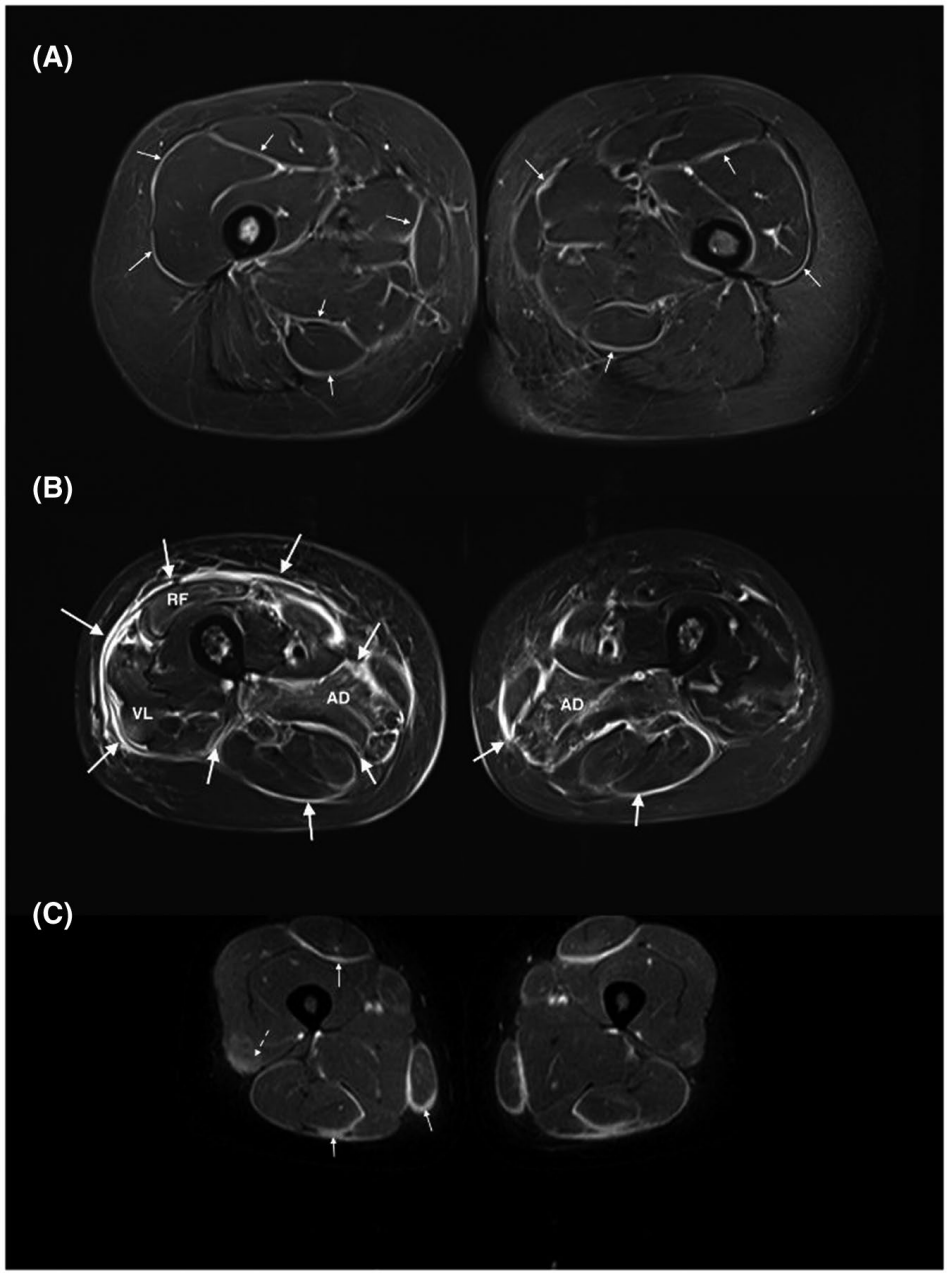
High fascia T2 signals in myositis: (A) patient with an eosinophilic fasciitis displays a diffuse hypersignal of the deep fascia and intermuscular septa (small arrows). The pelvic muscles (i.e., gluteus muscles) are also affected. (B) MRI of a patient with a graft versus host disease involving the fascia (big arrows) (diffuse hyperintensity and thickening of the deep fascia and intermuscular septa) and the muscles (especially both the adductor magnus muscles and the right quadriceps muscle). (C) ASyS patient dysplaying a fasciitis with hyperintense, thickened fascia and intermuscular septa on both sides with symmetrical distribution (full arrows). In addition, presence of a mild myositis attested by a blurred, slight T2 hyperintensity in the quadriceps muscles (dashed arrows). All pictures show thigh muscles MRI images (axial plane, T2 STIR w. seq). RF, rectus femori; VL, vastus lateralis
